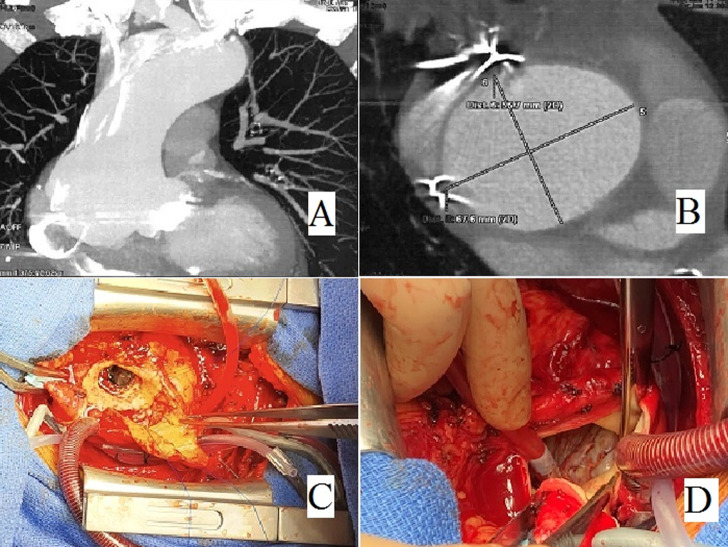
A) frontal section of a thoracic CT angiography showing a pseudoaneurysm of the tubular aorta; B) thoracic CT angiography in cross-section showing a 70 mm ectasia of the aortic root; C) intraoperative view showing a huge ectasia or pseudoaneurysm encompassing the right coronary sinus; D) intraoperative view showing perforation of the aorta by an epicardial electrode
